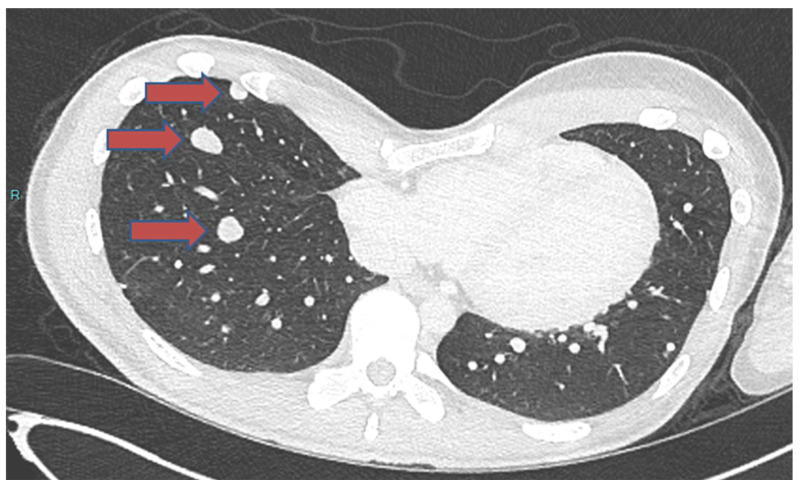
A chest CT scan showing the progression of metastasis before denosumab treatment. Red arrows indicate the nodules.
